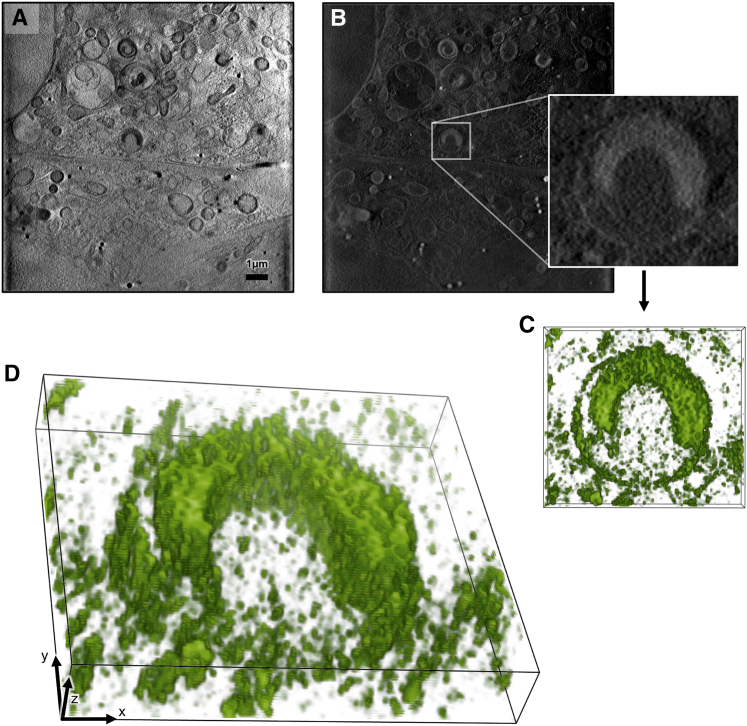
The 3D organization of virus-containing structures within cytoplasmic vesicles, related to Figure 6 (A and B) In (A), is a single Z axis slice of an ROI X-ray tomogram showing two infected cells at 2 h after infection (respective nuclei at top and bottom of left-hand side) and in (B) is the same with all pixel values inverted (necessary for volume rendering in Chimera) (Pettersen et al., 2004) with a sub-area expanded around a distinct semi-circular carbon-dense structure that is reovirus-positive. (C and D) In (C) is a surface rendering of a partial stack of the corresponding density looking down the Z axis and in (D), the same volume as (C), tilted to show the 3D structure. All images were generated with Chimera (Pettersen et al., 2004).
